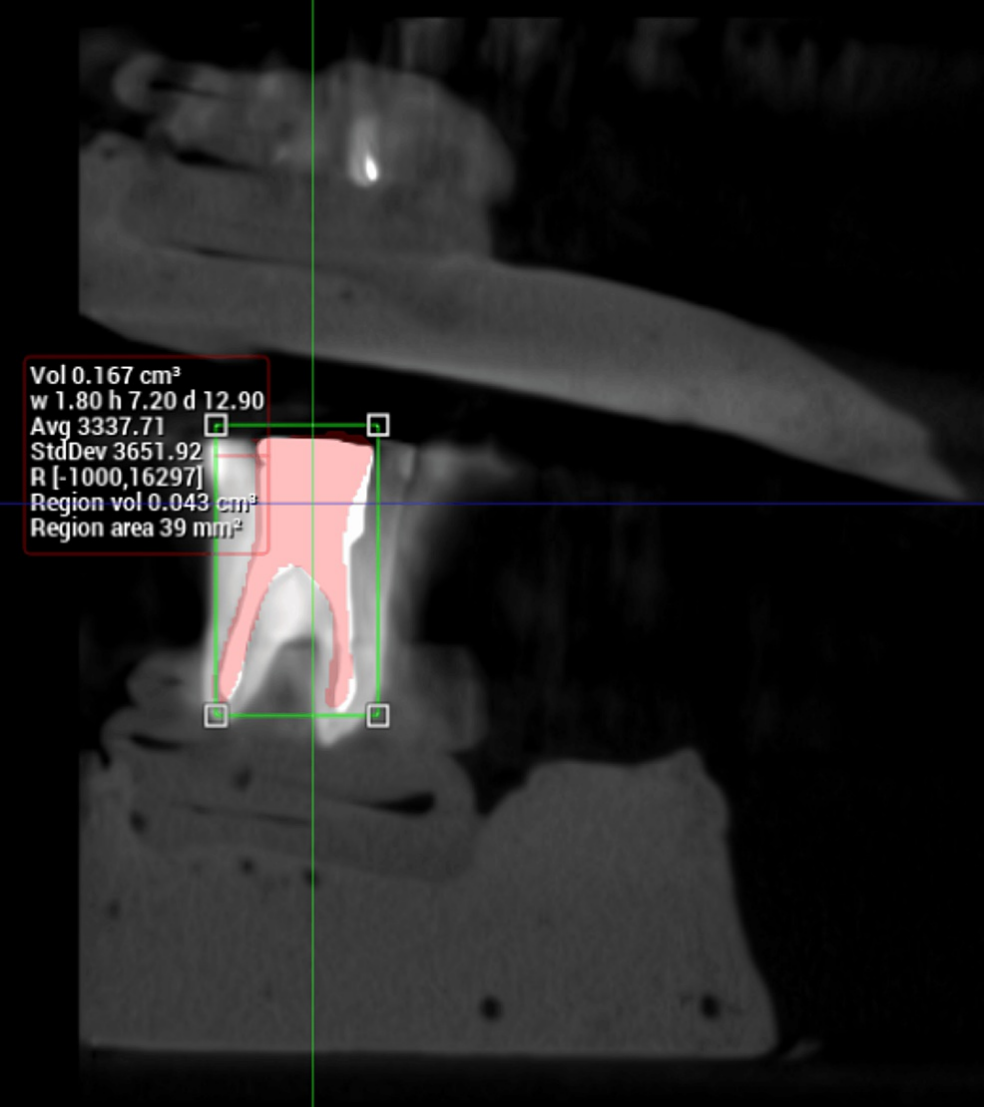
Calculation of volume from CBCT image taken after obturation CBCT: Cone beam computed tomography
